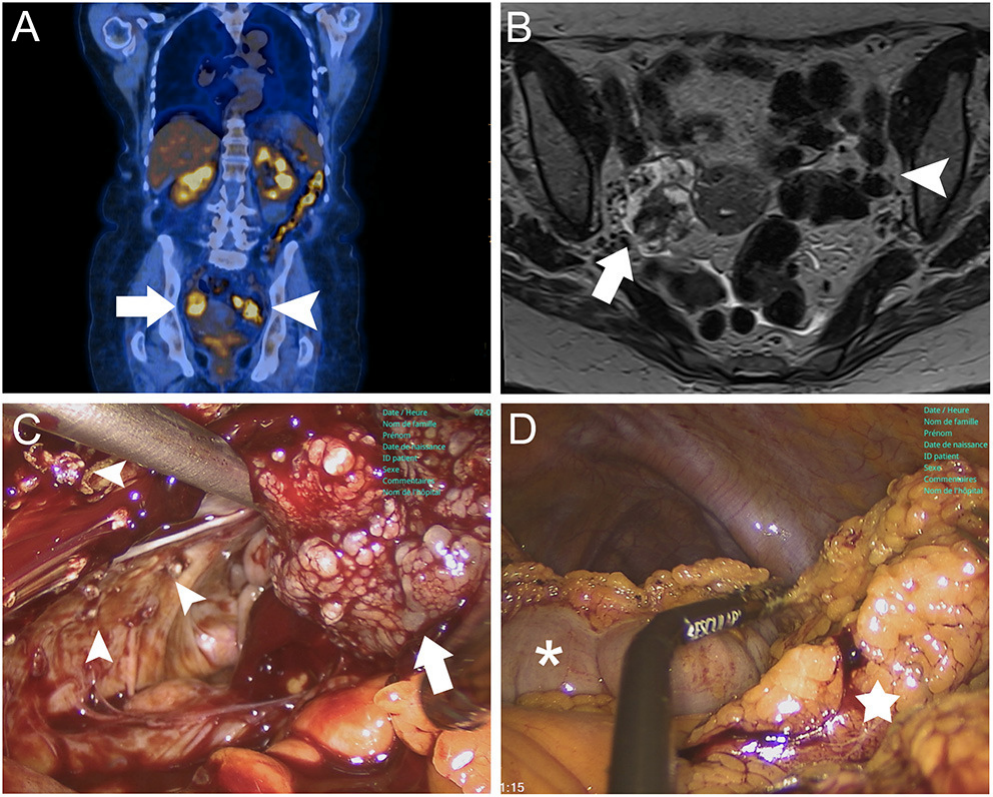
Case 1 images. (A) Coronal PET/CT scan demonstrating metabolically active lesions on right (white arrow) and left ovaries (white arrowhead). (B) Axial gadolinium-enhanced T1-weighted MRI showing bilateral adnexal masses with heterogeneous solid appearance, irregular contours, and measuring 4.1 × 2.9 × 4.2 cm on the right (white arrow) and 3.1 × 1.4 × 1.0 cm on the left side (white arrowhead). (C) vNOTES approach showing a slightly enlarged right ovary with external vegetations (white arrow) and multiple peritoneal implants on the right pelvic sidewall (white arrowheads). (D) vNOTES infracolic omentectomy with separation of the greater omentum (white star) from the transverse colon (white asterisk) using a 5-mm Caiman^®^ grasper.
